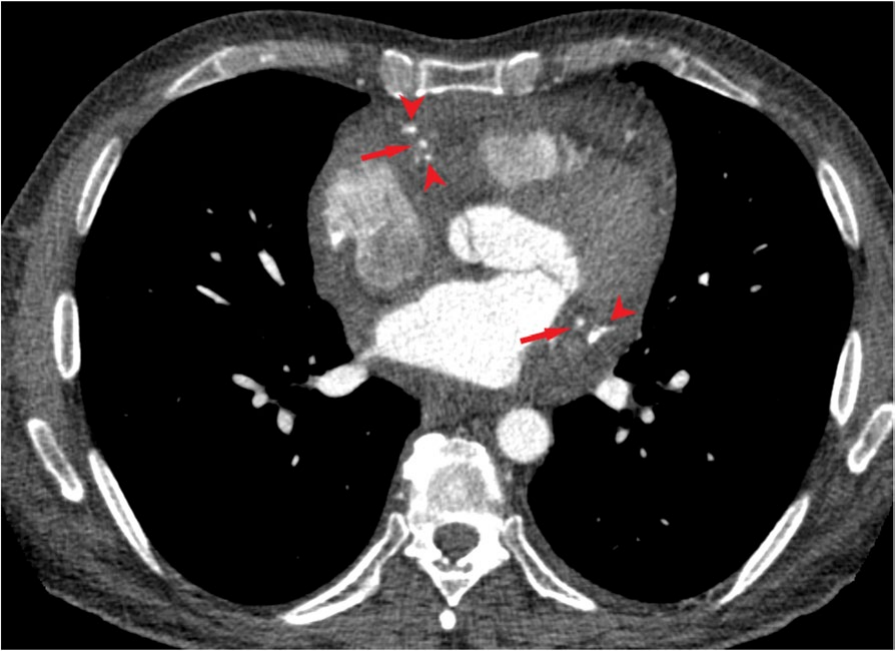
Contrast enhanced chest CT scan shows periarterial soft tissue infiltration around the coronary arteries (arrow) with foci of calcification (arrowhead)
